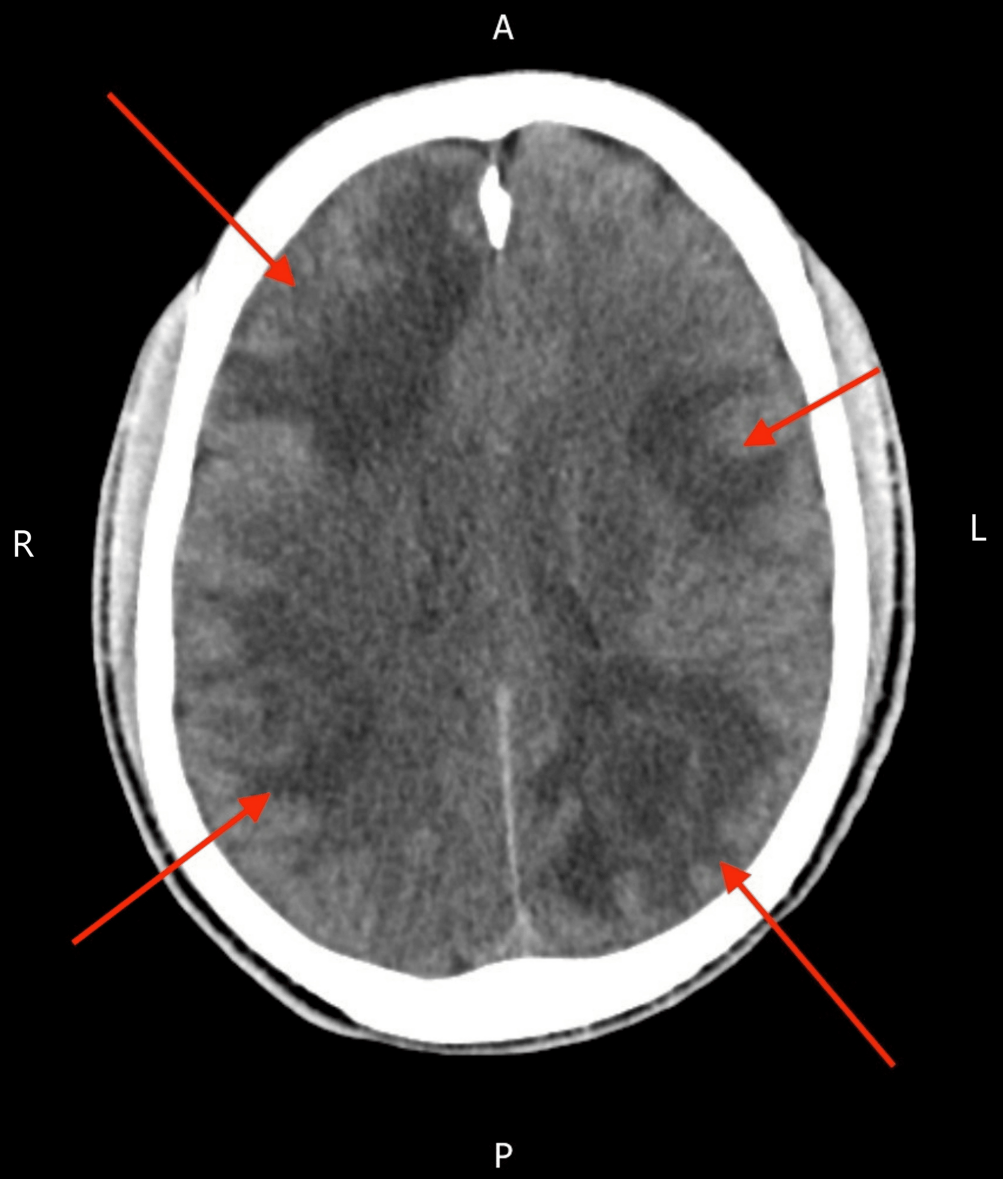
Non-contrast CT of the head showing multifocal oedema throughout both cerebral hemispheres with sulcal effacement CT: computed tomography
